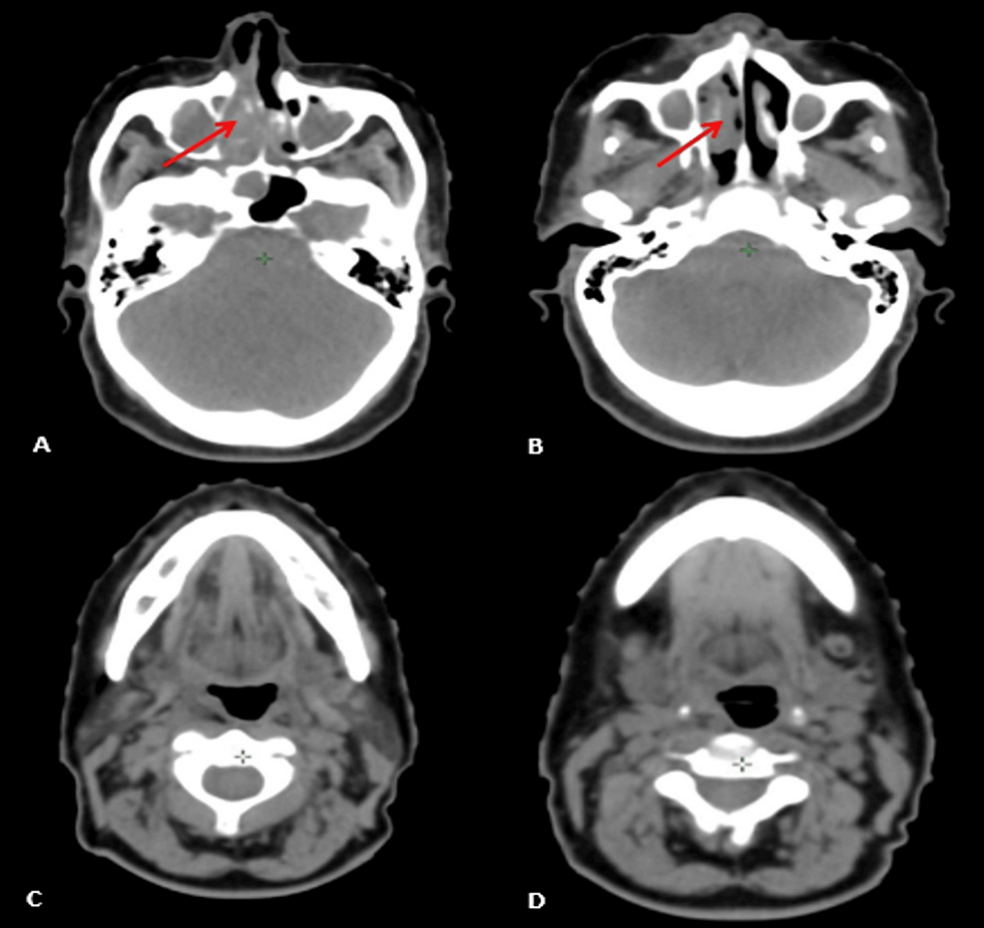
Computed tomography scan images Post-chemotherapy scan images A, B: Axial view showing the reduction of the right nasal mass (red arrow). C, D: Axial view showing the reduction in cervical lymphadenopathy
